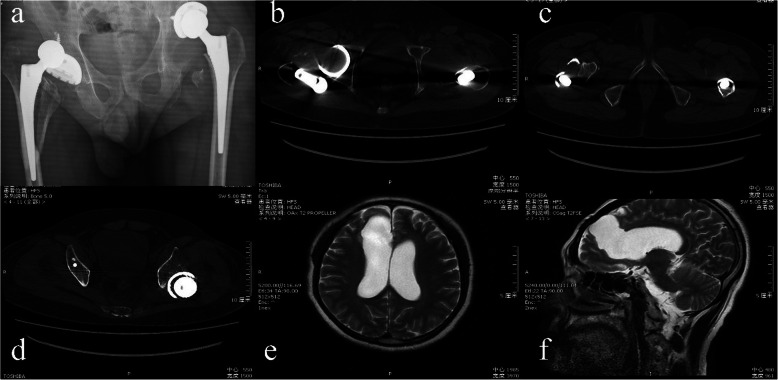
The anterior-posterior radiograph of the bilateral hip (a) showed dislocation of the right femoral head, displaced oblique fracture of the right proximal femur involving greater trochanter, upward dislocation of the left hip joint involving acetabular endoprosthesis components, fracture of the right acetabular and avulsion fracture of right lesser trochanter; computed tomography scan showed upward and backward dislocation of the right femoral head (b), displaced oblique fracture of the right proximal femur involving greater trochanter (c) and upward dislocation of the left hip joint involving acetabular endoprosthesis components (d); MRI showed a large encephalomalacia focus (4 cm × 3.5 cm × 3 cm size) located at the right frontal lobe and communicating with the lateral ventricle (e, f)
